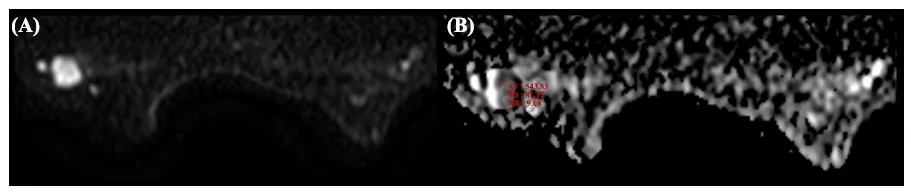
Diffusion-weighted imaging and ADC mapping of axillary lymph nodes. (A) DWI (b = 800 s/mm^2^) showing high signal intensity in a metastatic lymph node, indicating diffusion restriction. (B) Corresponding ADC map with ROI placement demonstrating low ADC value (747 ×10^−3^ mm^2^/s) in the same metastatic node.
